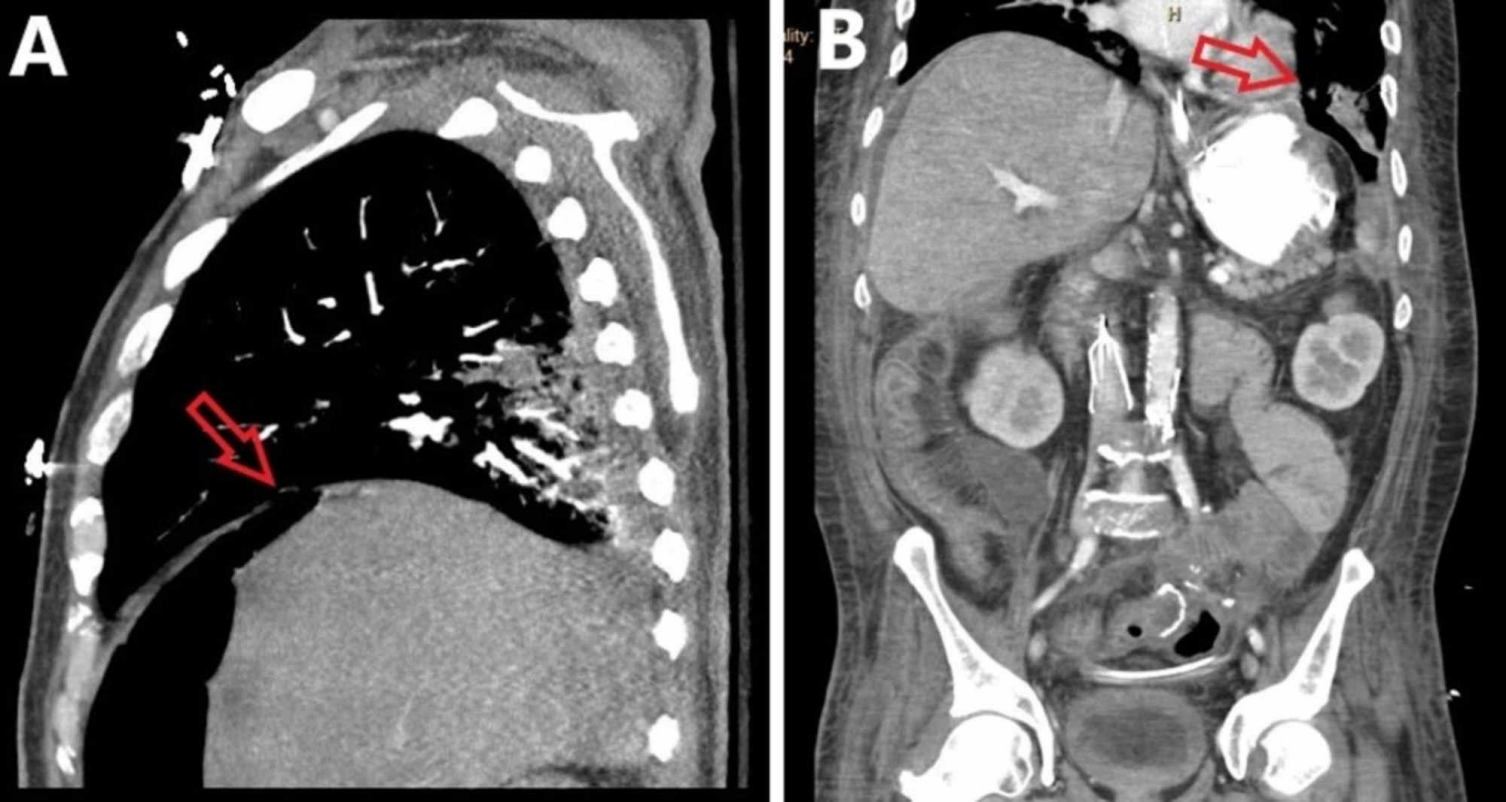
Contrasted computed tomography of the chest (A) and the abdomen and pelvis (B). (A) Sagittal plane computed tomography of the chest demonstrating a left bronchoperitoneal fistula (red arrow). (B) Coronal plane computed tomography of the abdomen and pelvis demonstrating a left bronchoperitoneal fistula (red arrow).
